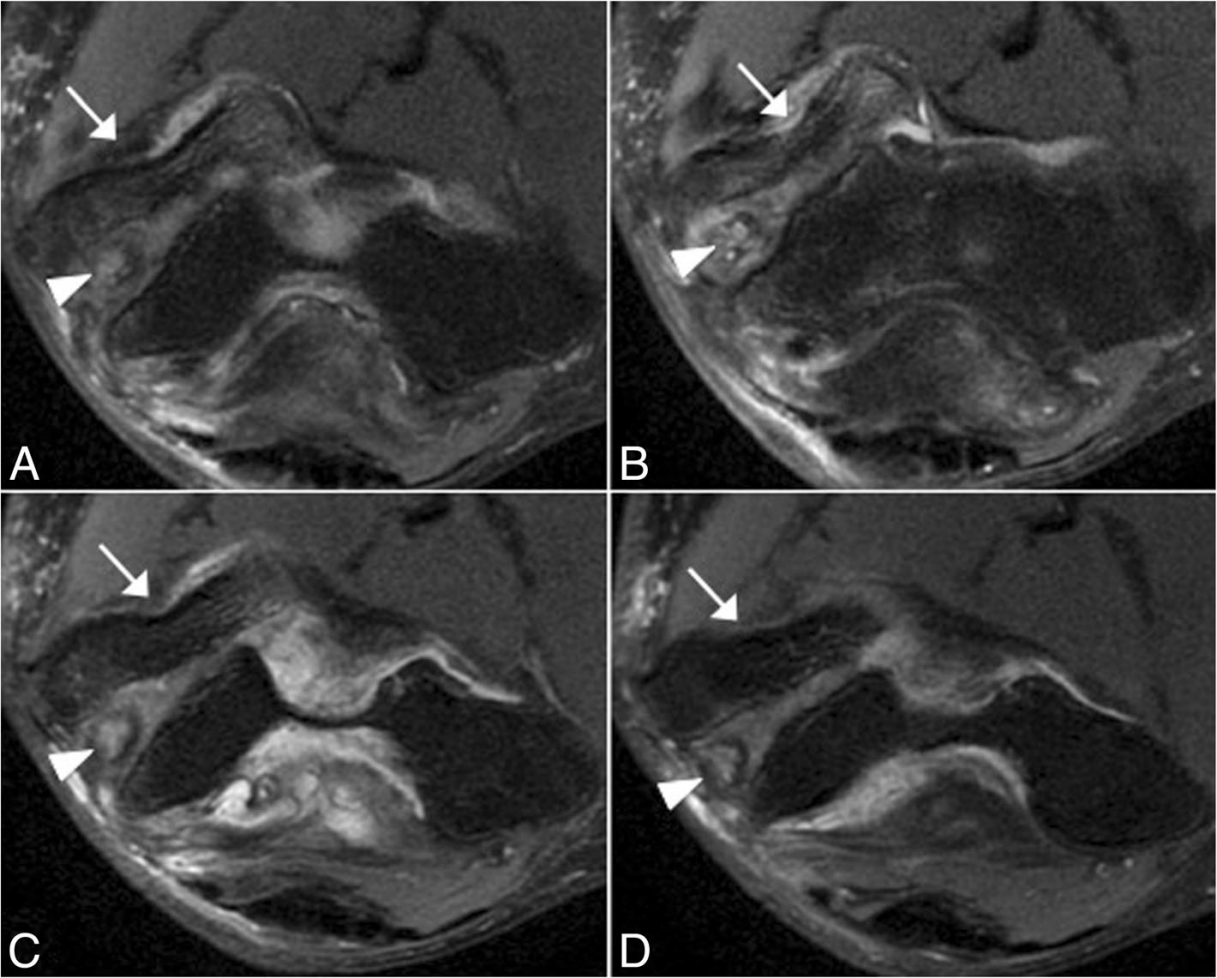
A 45-year-old woman with pain, muscle weakness, and paresthesias after a fall on an outstretched hand. Consecutive axial FS PD-weighted MRI (a–d) showing acute avulsion fracture of the medial epicondyle (white arrows). The ulnar nerve is trapped between osseous fragments (white arrowheads), showing intraneural increased signal intensity
